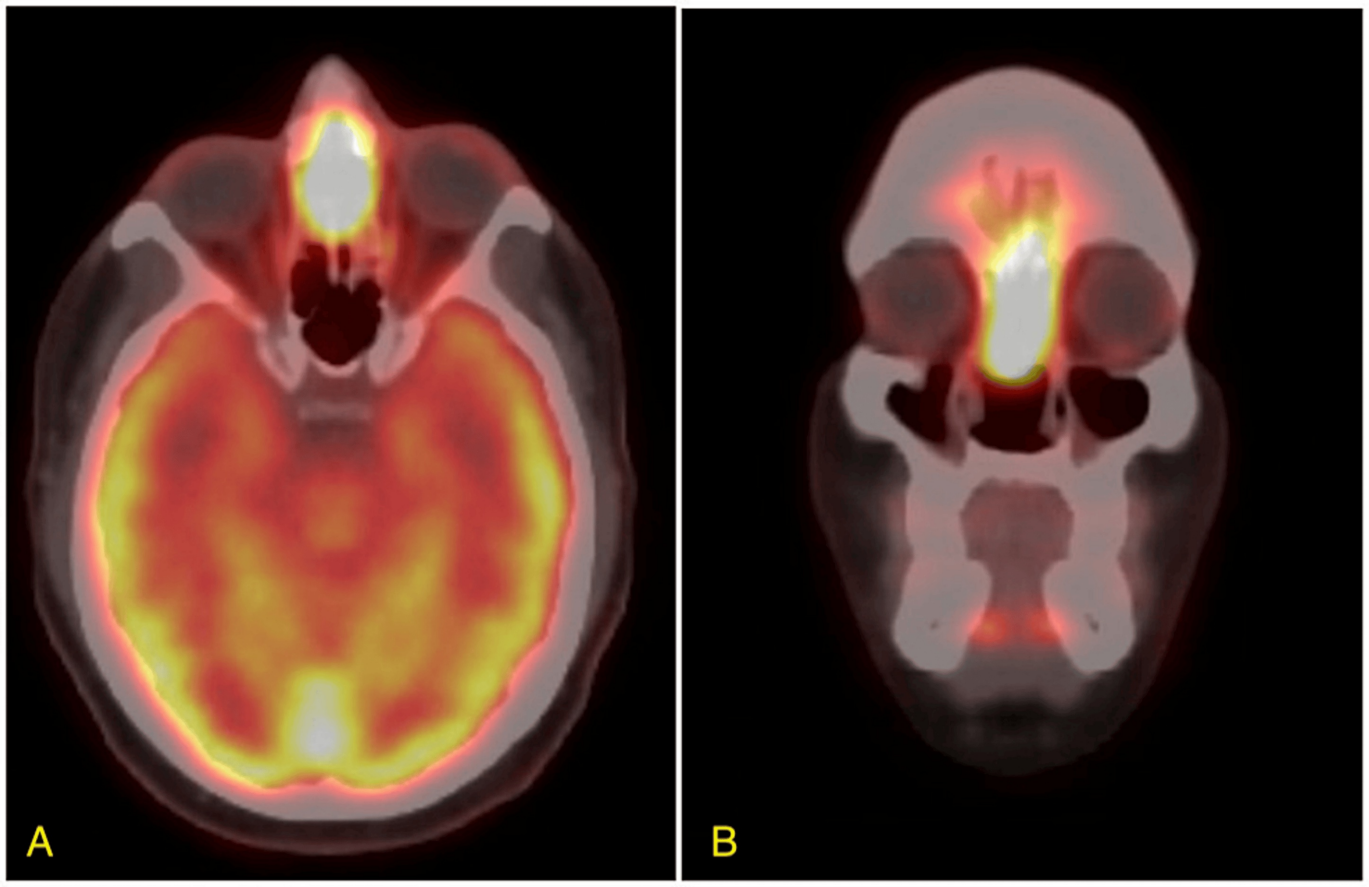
Pre-treatment axial (A) and coronal (B) PET/CT images
